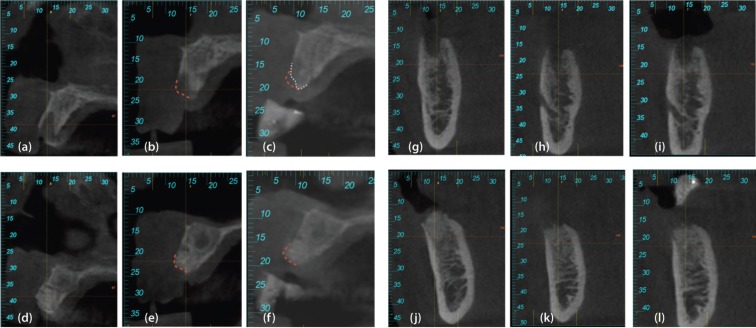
Case 1, left. (a) CBCT section of the baseline control socket (immediately post-surgery). (b) CBCT section of the control socket at 8 weeks. (c) CBCT section of the control socket at 16 weeks. (d) CBCT section of the baseline ADM socket (immediately post surgery). (e) CBCT section of the ADM socket at 8 weeks. (f) CBCT section of the ADM socket at 16 weeks. Case 2, right. (g) CBCT section of the baseline control socket (immediately post surgery). (h) CBCT section of the control socket at 8 weeks. (i) CBCT section of the control socket at 16 weeks. (j) CBCT section of the baseline ADM socket (immediately post-surgery). (k) CBCT section of the ADM socket at 8 weeks. (l) CBCT section of the ADM socket at 16 weeks.
